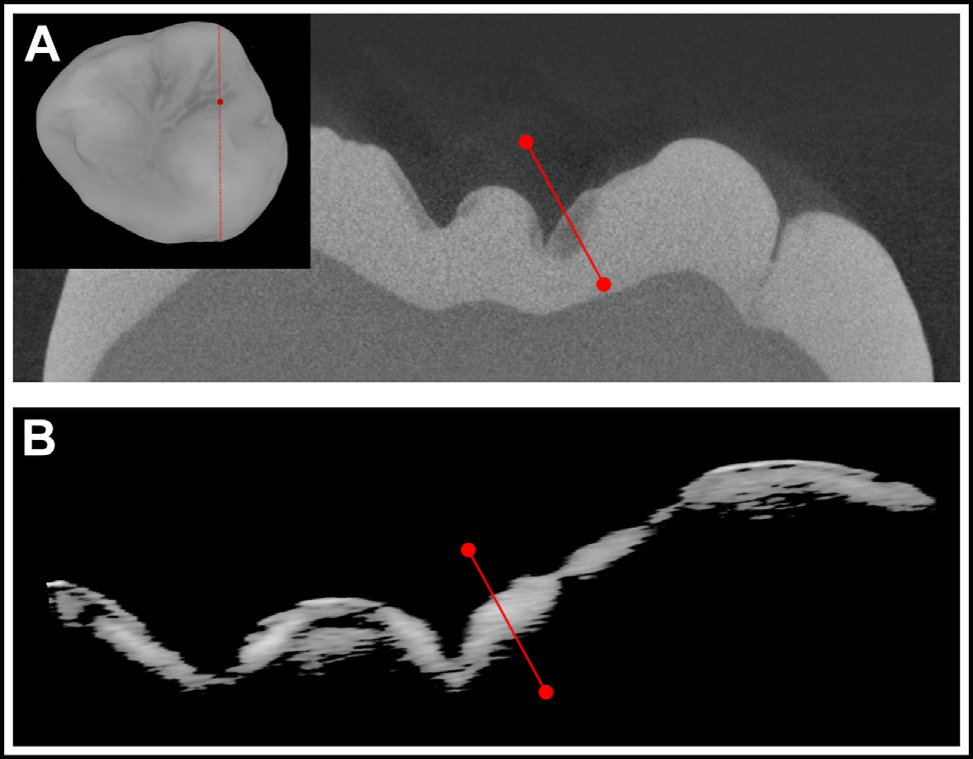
Microcomputed tomographic surface rendering of the occlusal surface of a tooth with occlusal lesions showing the position at which the microcomputed tomographic (A) and processed optical coherence tomographic (B) cross-sectional images shown were extracted from the position of the dotted red line. The solid red lines show the position that was chosen for analysis.
